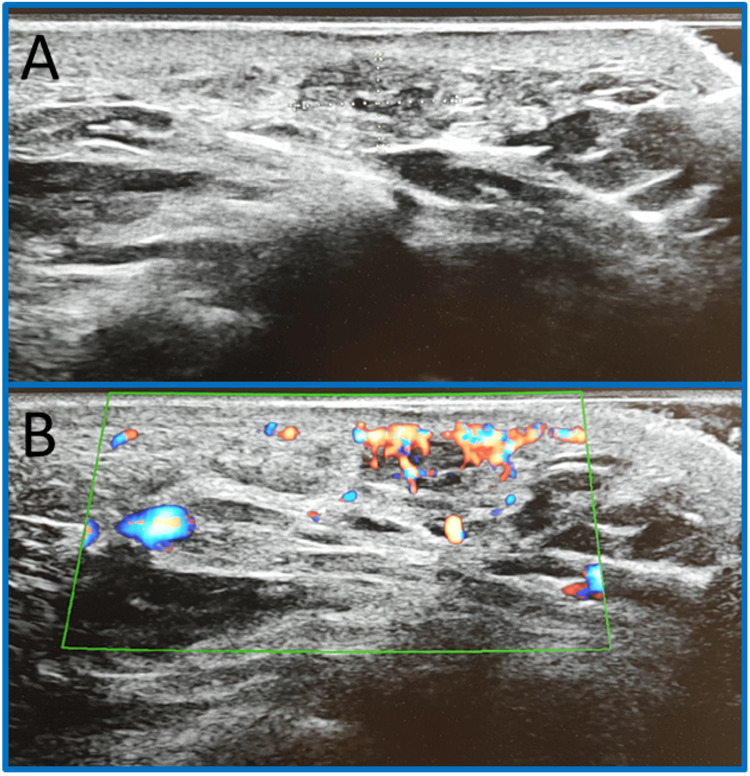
Lesion on longitudinal ultrasound (A) with Doppler showing venous flow (B), suggestive of hemangioma
